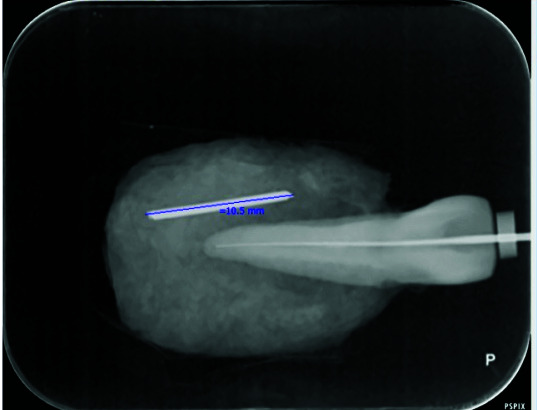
Normal digital radiograph without any software enhancement
